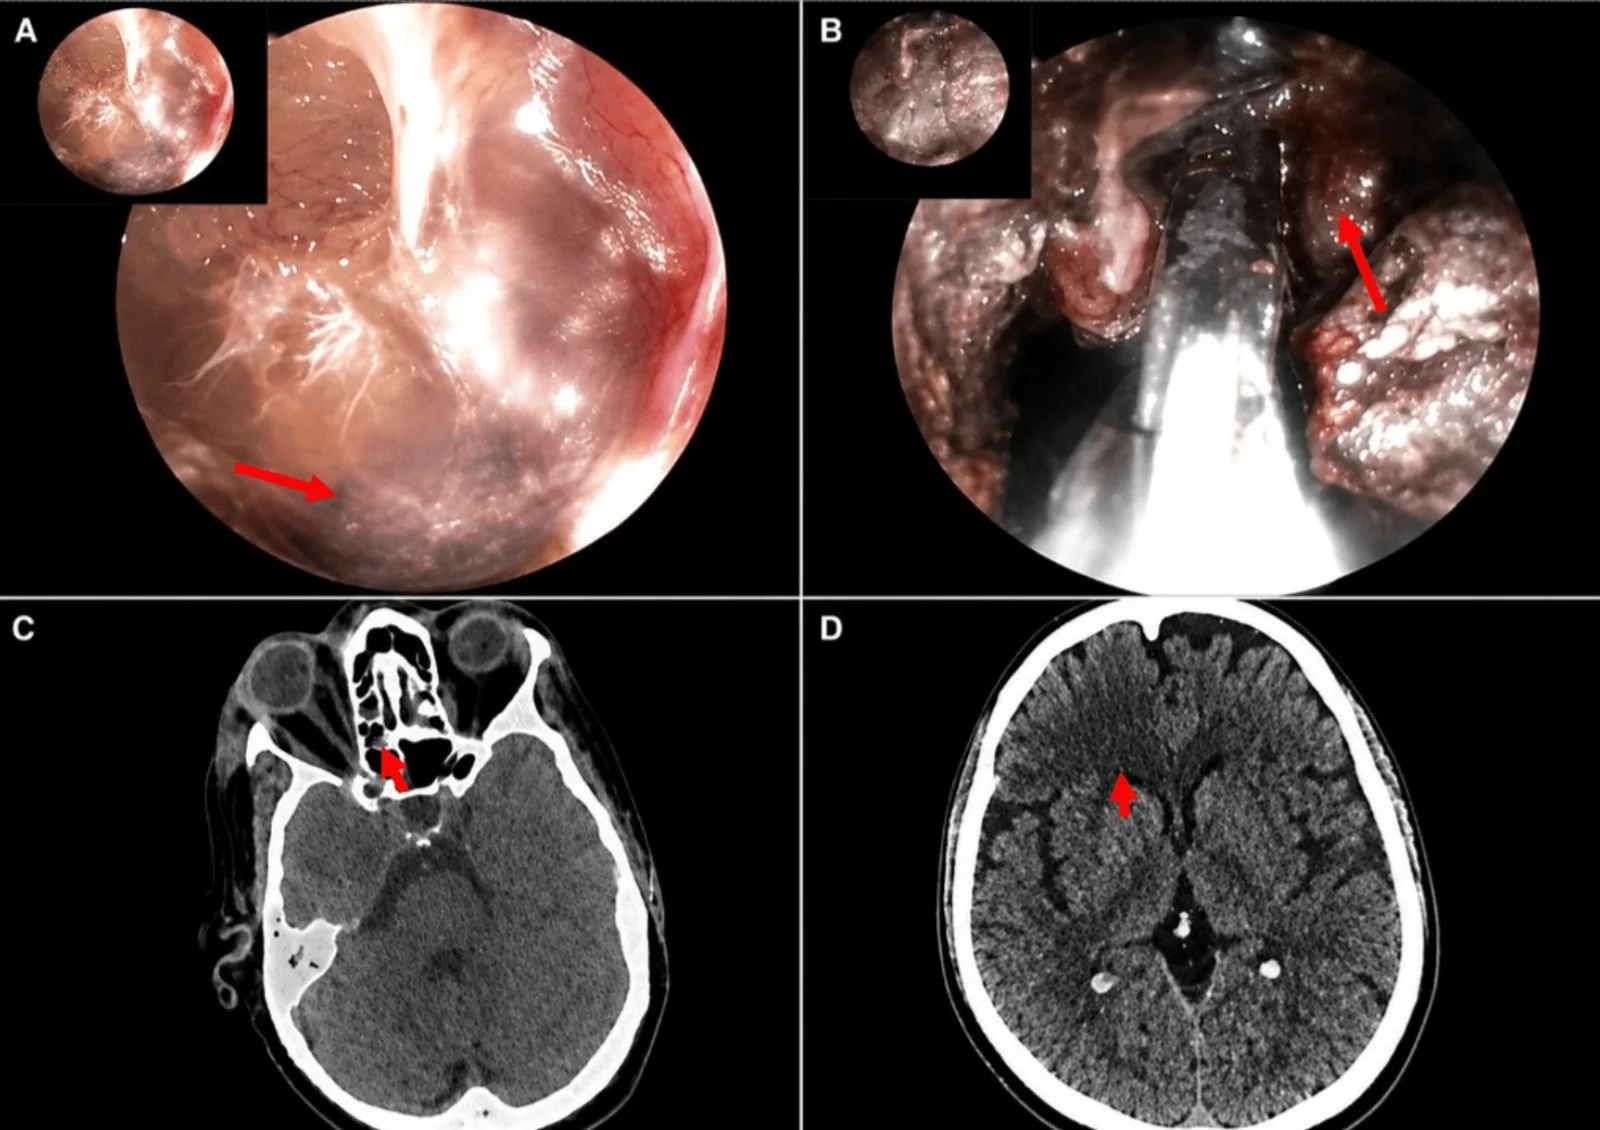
Endoscopic and CT findings in rhino-orbito-cerebral fungal Infection (A) Nasal endoscopy demonstrating necrotic debris involving the right nasal turbinate (arrowhead); (B) Endoscopic debridement of necrotic tissue from the right nasal cavity (arrowhead); (C) Axial computed tomography of the paranasasal sinuses showing opacification of the ethmoid and sphenoid sinuses with extension into the right orbit (arrowhead); (D) Axial non-contrast brain computed tomography demonstrating an irregular hypodense area in the right frontal lobe, suggestive of cerebral edema (arrowhead).
